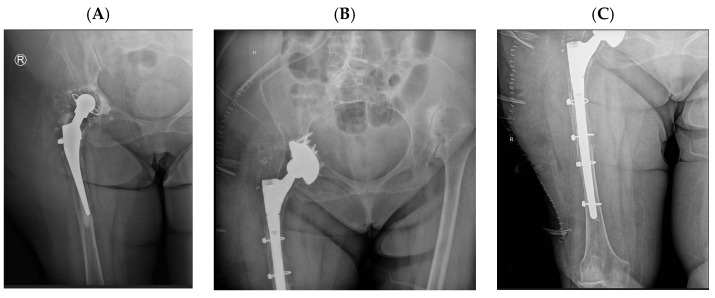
(A) Radiograph showing a right THA with a cemented acetabular component and femoral stem, complicated by femoral stem loosening and partial acetabular cup loosening. (B,C) Postoperative radiographs following revision surgery.
